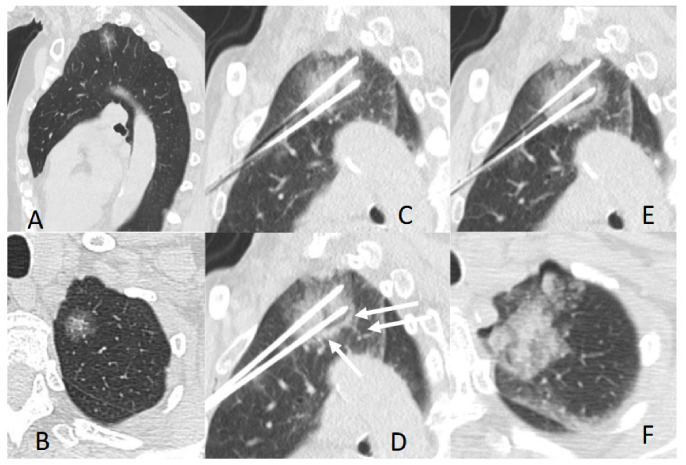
(A,B) Sagittal and axial CT scan images of biopsy-proven left apical adenocarcinoma with lepidic pattern with a maximum diameter of 28 mm in the craniocaudal dimension. (C) A sagittal reconstruction image with two cryoprobes placed at the edges of the tumor approximately 2 cm apart (1-2 rule). The current scan is at end of a 3 min freeze cycle. The ice ball is not seen during the freeze cycle and is best visualized during the thaw cycle. (D) An image following 3 min of thaw cycle. The edge of the melting ice ball is seen as a hemorrhage and a rim of consolidation (arrow). (E) Cryoablation at the completion of 3 min freeze, 3 min passive thaw, 5 min freeze, 3 min passive thaw, 7 min of freeze. (F) Following completion of cycles, active thaw was performed and the probes were removed. Post-ablation hemorrhage is surrounding the primary tumor.
